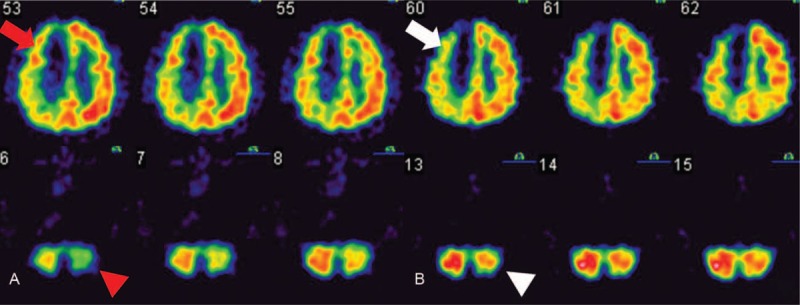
Regional perfusion SPECT before and after intravascular laser irradiation. The 2 SPECT images show the regional perfusion. The white to red area indicated better perfusion than the blue and green area. (A) This regional perfusion SPECT (conducted on poststroke day 14) shows that the infarction region (indicated by red arrow) covers the territory of the anterior cerebral artery on the right hemisphere and decreased perfusion on the left cerebellar hemisphere (indicated by red arrow head). (B) The second regional perfusion SPECT (conducted on poststroke day 63) still showed hypoperfusion in the right cerebral hemisphere (indicated by white arrow), but more blood flow in the left cerebellar hemisphere (indicated by white arrow head). SPECT = single-photon emission computed tomography.
